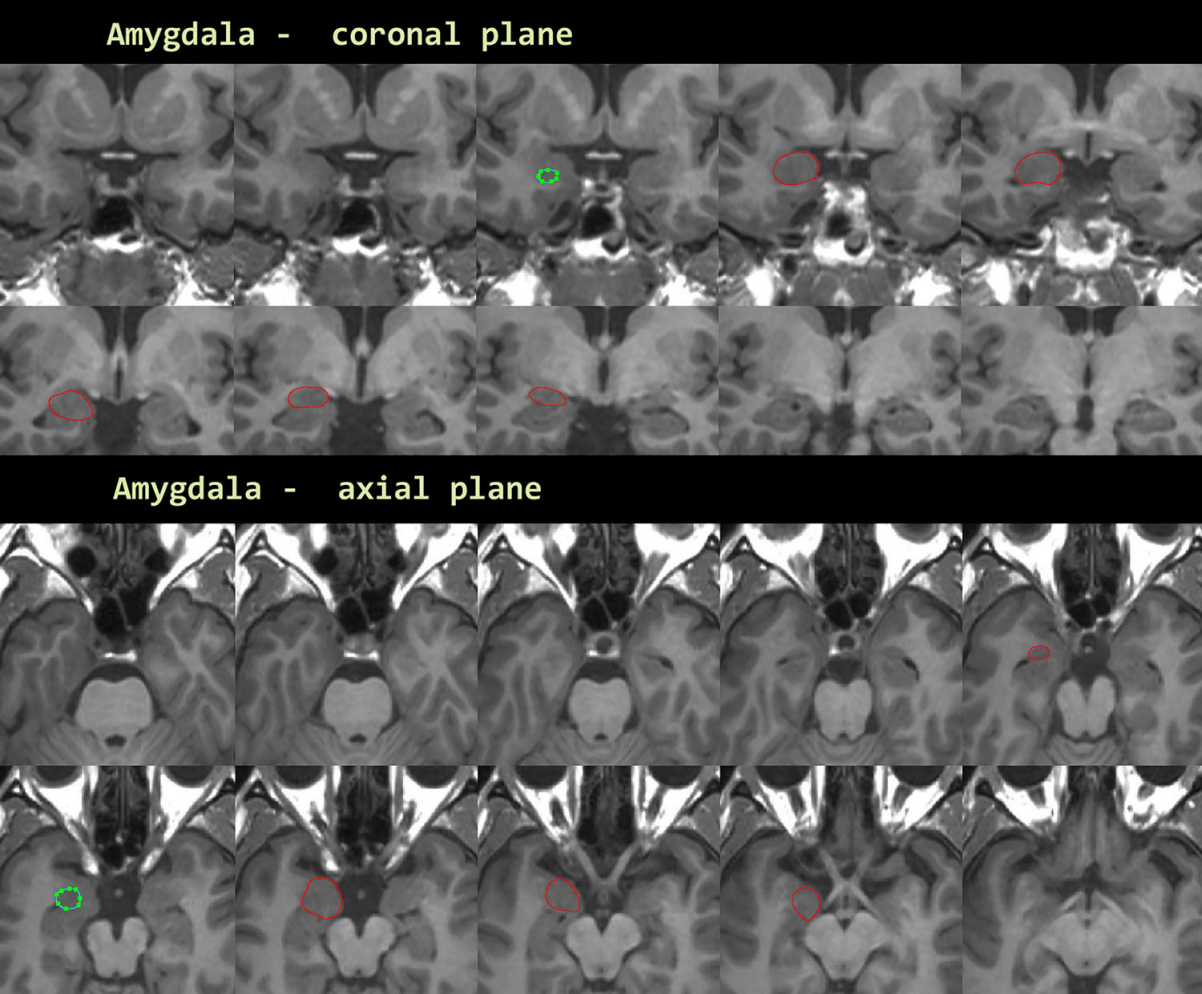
Snapshot from JIM8 screen that presents for one individual, the manual segmentation for right amygdala volume measurement in two planes coronal (above) and axial (below) under the instruction of an experienced neuro‐radiographer.
